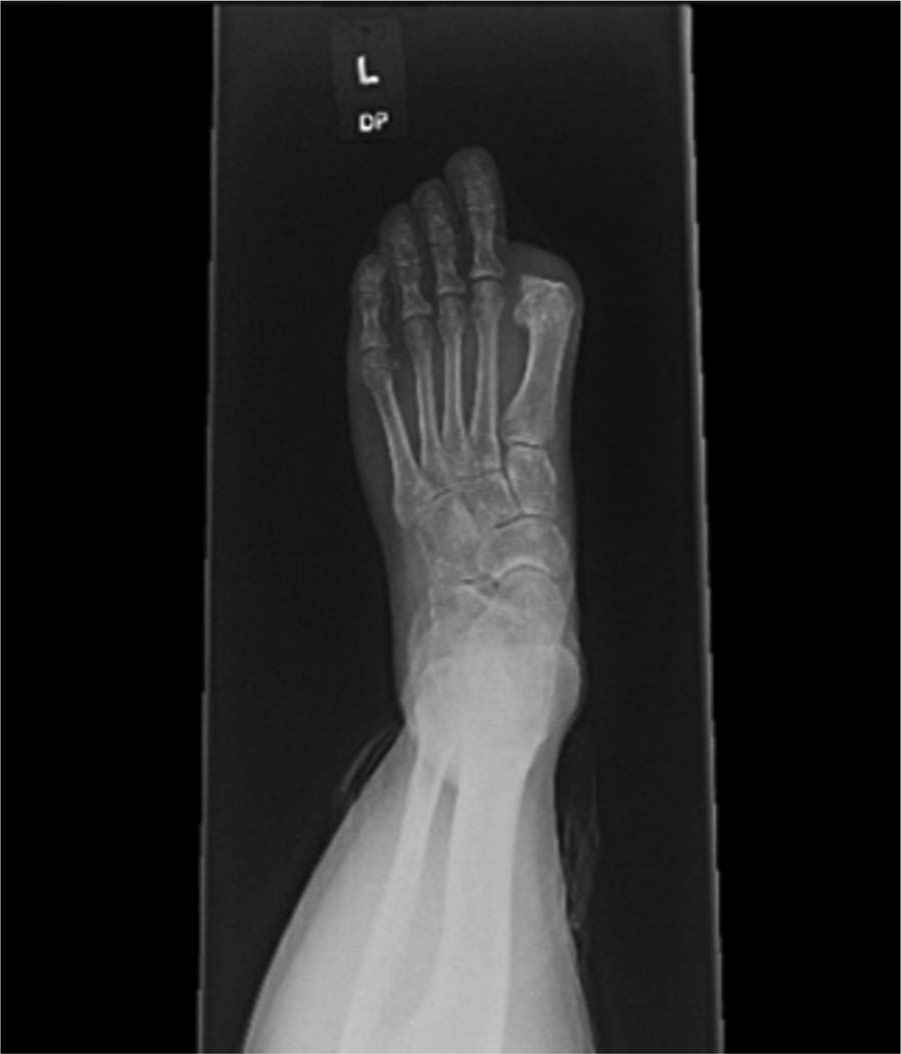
Radiographs at one year demonstrating satisfactory healing.
